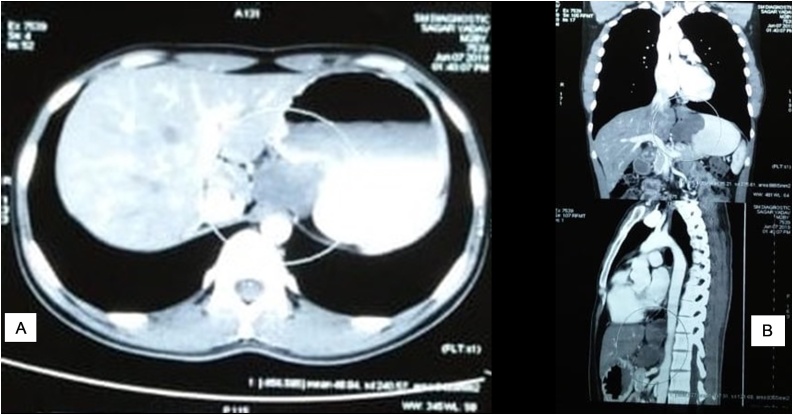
(A&B): CT scan showing the oesophageal lesion.
